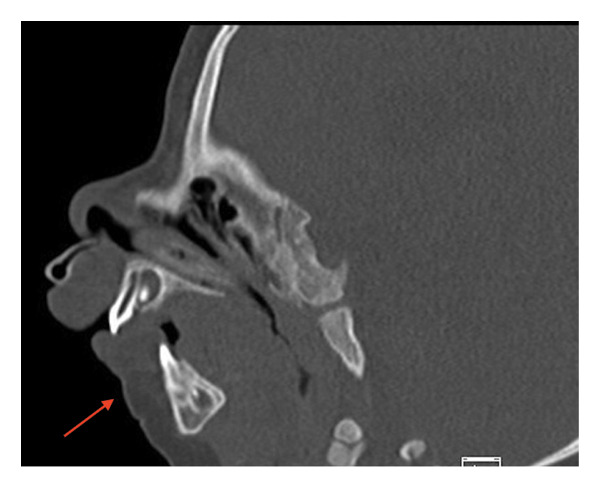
CT scan of facial bones at 10 months of age revealing overall improvement despite recent regression in micrognathia. The improved micrognathia is indicated with an arrow.
